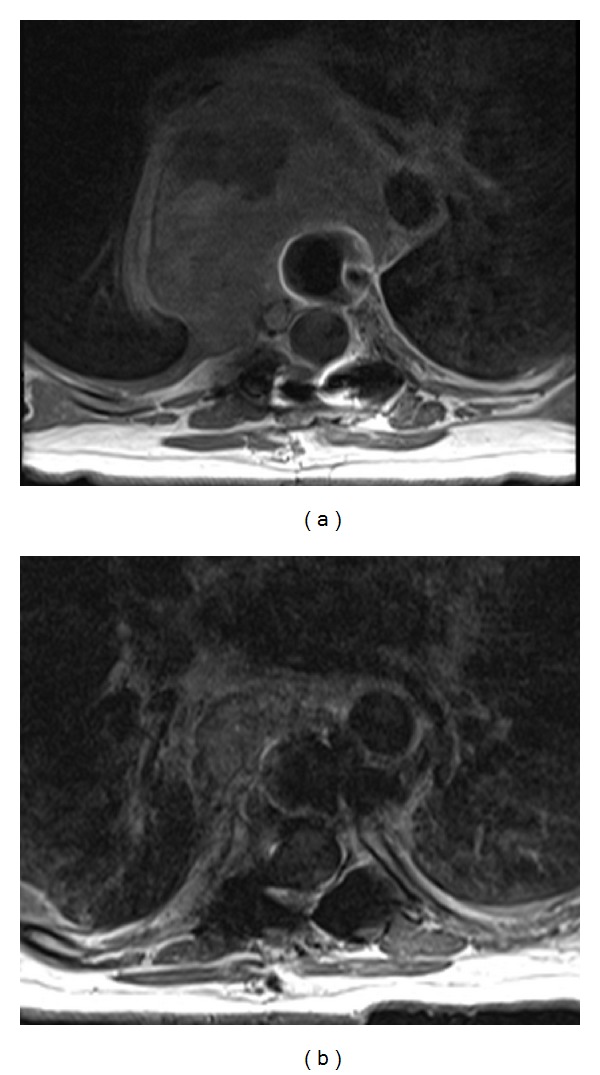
Axial T1 weighted postcontrast computed tomographic images show a large mid-thoracic spine mass (a) before and (b) 10 months after denosumab therapy.
